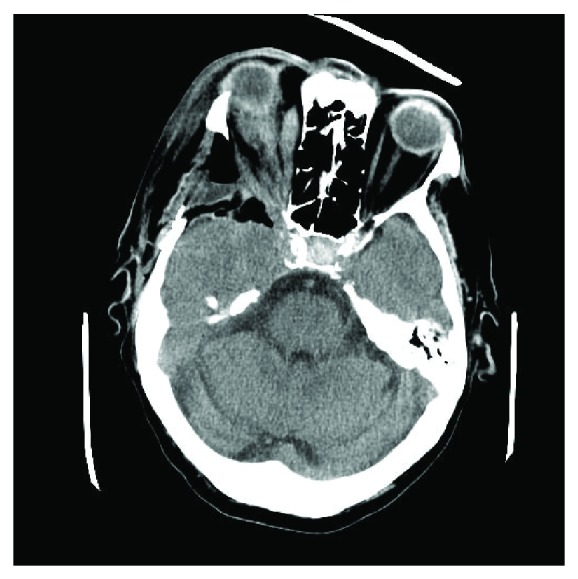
Axial post-operative CT scan demonstrating orbital decompression.
